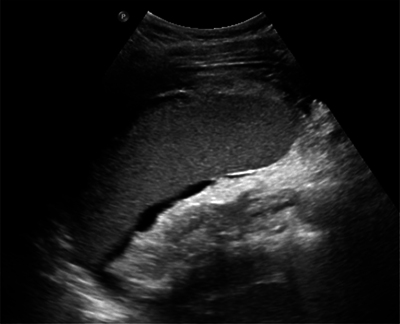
Abdominal ultrasound shows splenomegaly and a perisplenic, layered fluid collection.
